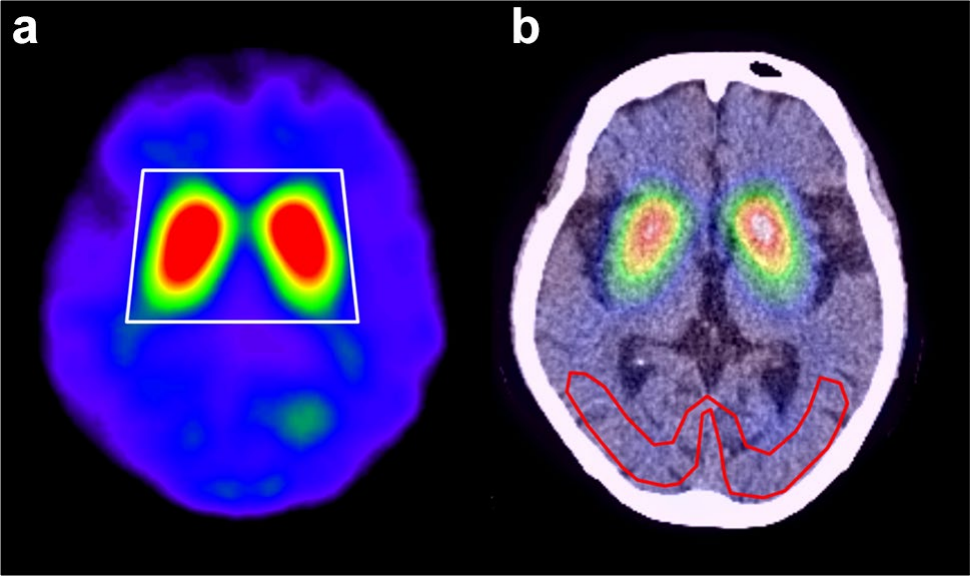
Striatal trapezoid VOI (VOI_t_) location at the slice level of the striatal centre on DaT-SPECT (a). The VOI_t_ includes the whole striatum for both sides. To obtain the background activity, the background VOI (VOI_bg_) was drawn on the occipital cortex using several image slices avoiding the sinus region and the cerebrospinal space (b)
